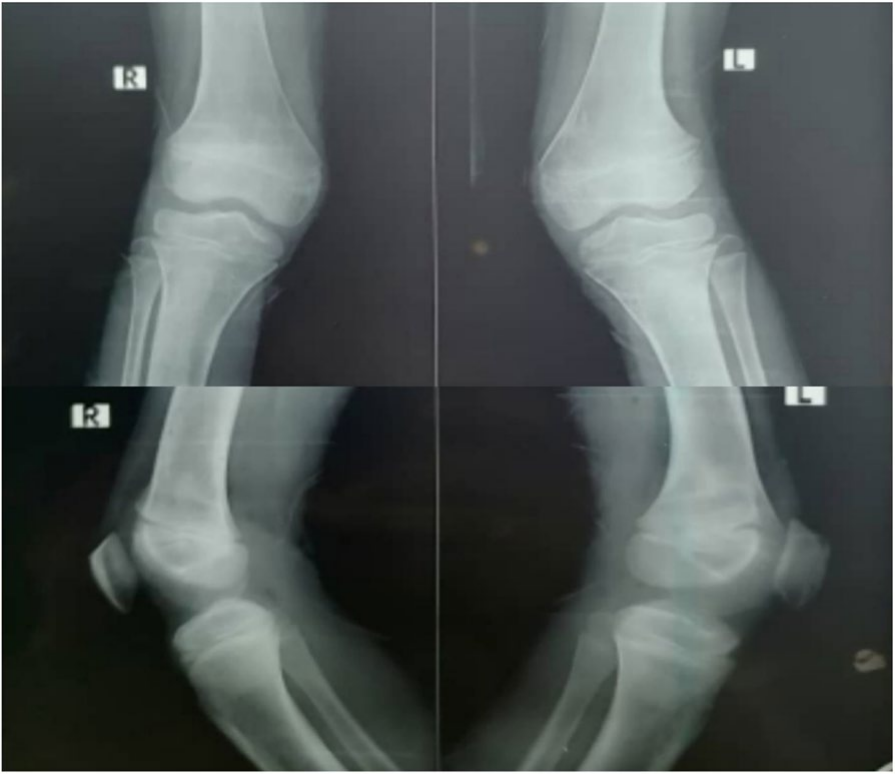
X-ray bilateral knees shows improvement of osteopenia and healing of rickets signs after surgery
